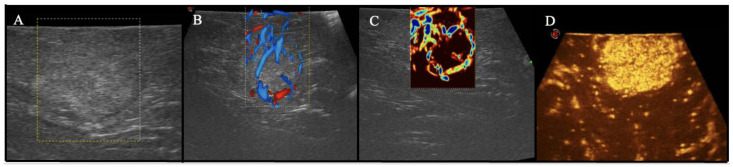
IOUS and iCEUS visualization of HBs: (A) standard B-mode examination showing HBs appear as hyperechoic lesions; (B) color Doppler used to evaluate the HBs’ perfusion pattern; (C) micro-V Doppler allows to obtain a micro-vascular perfusion analysis, depicting the surrounding vessels and the smaller capillaries inside the lesion; (D) iCEUS visualization showing a rapid and homogeneous enhancement of the nodular part.
